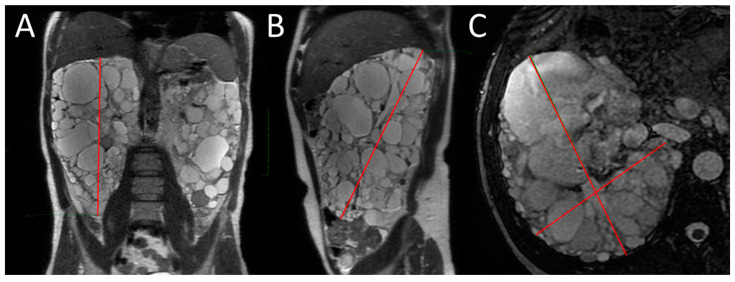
MRI measurement of renal diameters on the right kidney of a patient with ADPKD for SKV computation using the ellipsoid method. Coronal length (A), sagittal length (B), width and depth (C).
